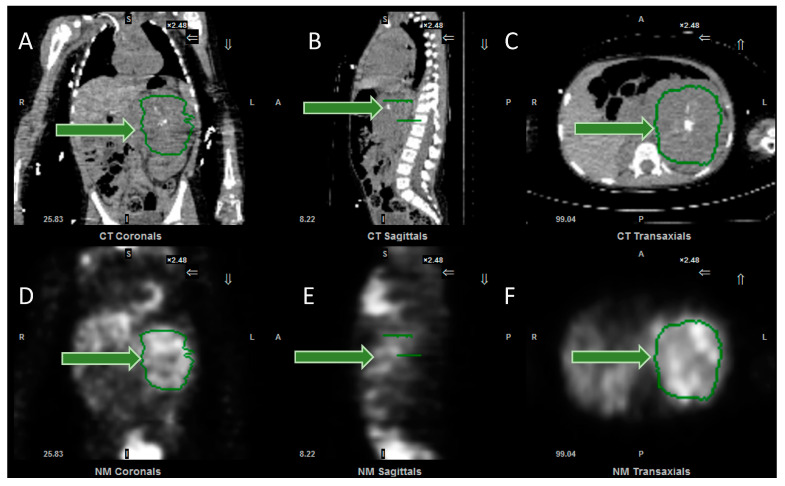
Images from the software to measure SPECT SUV (standardised uptake values). (A) Coronal, (B) sagittal and (C) axial CT images, together with (D) coronal, (E) sagittal and (F) axial SPECT images, demonstrating the tumour, outlined in green (arrowed). Following input of data, including administered activity and organ delineation, the system will calculate the minimum, maximum and mean (standard deviation) of the SUV, expressed as g/mL. Same patient as in Figure 1.
